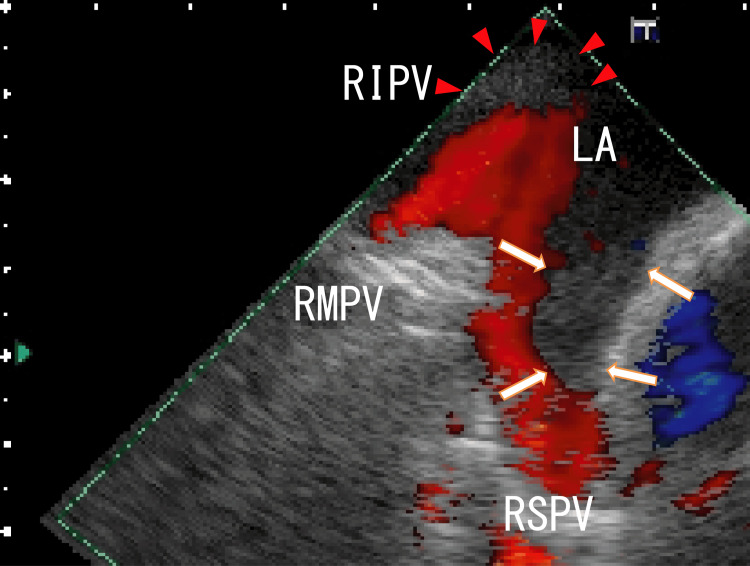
TEE images showing thrombi in the left atrium Transesophageal echocardiography (TEE) images showing expanded left atrium (LA) thrombi from the right superior pulmonary vein (RSPV), and these thrombi were dark masses that appeared to be adhered to the wall of the RSPV and the LA. Thrombi were identified as a lack of blood flow from the RSPV (arrows). The thrombi had no white areas at all. Blood flows from the RSPV and right middle pulmonary vein (RMPV) were shown as red areas. Thrombi expanded from the right inferior pulmonary vein (RIPV) and were depicted as dim white areas (arrowheads). Left atrium diverticula (LADs) were not detected.
